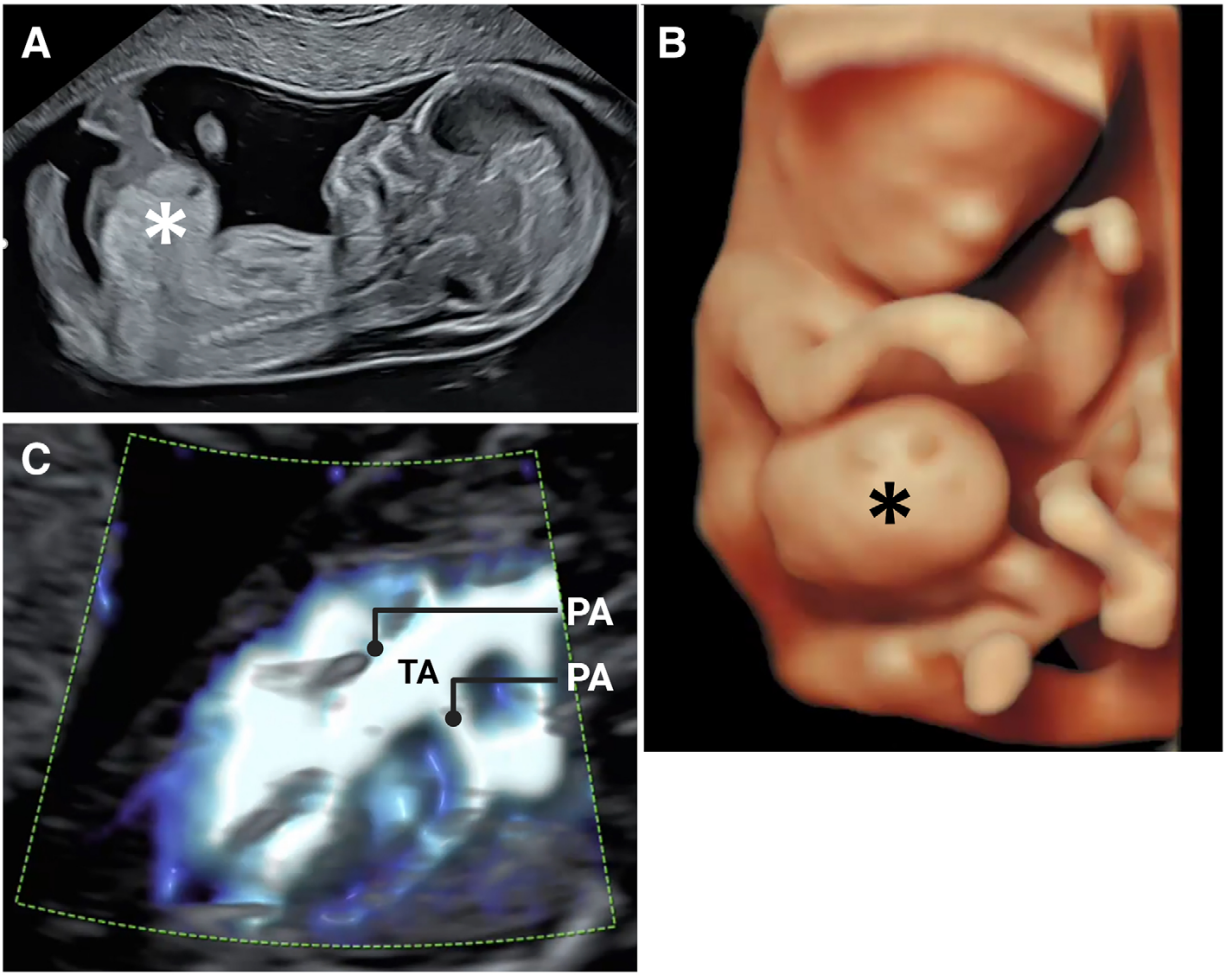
A complex case of truncus arteriosus with omphalocele, diagnosed at 12^+6^ weeks. The omphalocele (*) is imaged in the longitudinal plane (A) and with 3D rendering (B). The truncus arteriosus (Type II) is demonstrated with the Slow*flow*HD ultrasound modality (C) (PA, pulmonary arteries; TA, truncus arteriosus).
